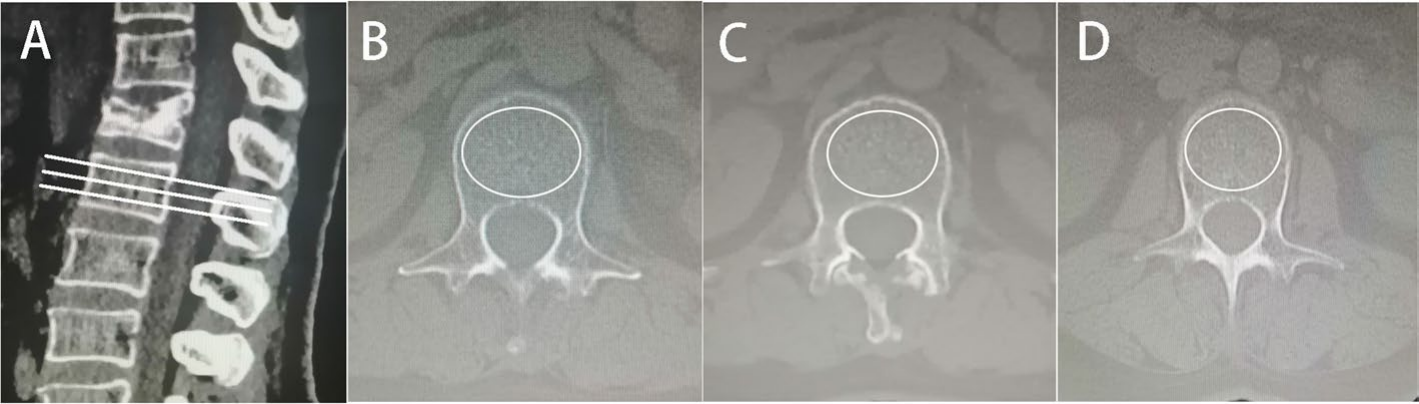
CT values were measured by PACS. A is a sagittal image of the lumbar spine with three tangents made on the measured vertebrae, corresponding to the three levels of (B), (C) and (D) in the axial position. The largest elliptical region of interest (ROI) containing only bone trabeculae was drawn in the axial position to obtain the average Hu value
